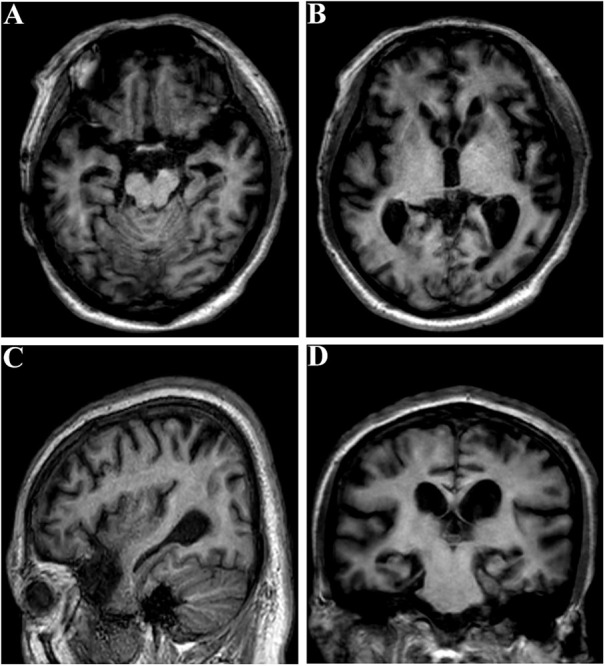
Brain MRI of the proband (family 1, III-3) at the age of 43. (A–C) Global brain atrophy especially in the temporal region and hippocampus. (D) Widened choroid fissure and temporal horn dilation, hippocampal atrophy. The scale for median temporal lobe atrophy (MTA-scale) was 2–3 degree.
